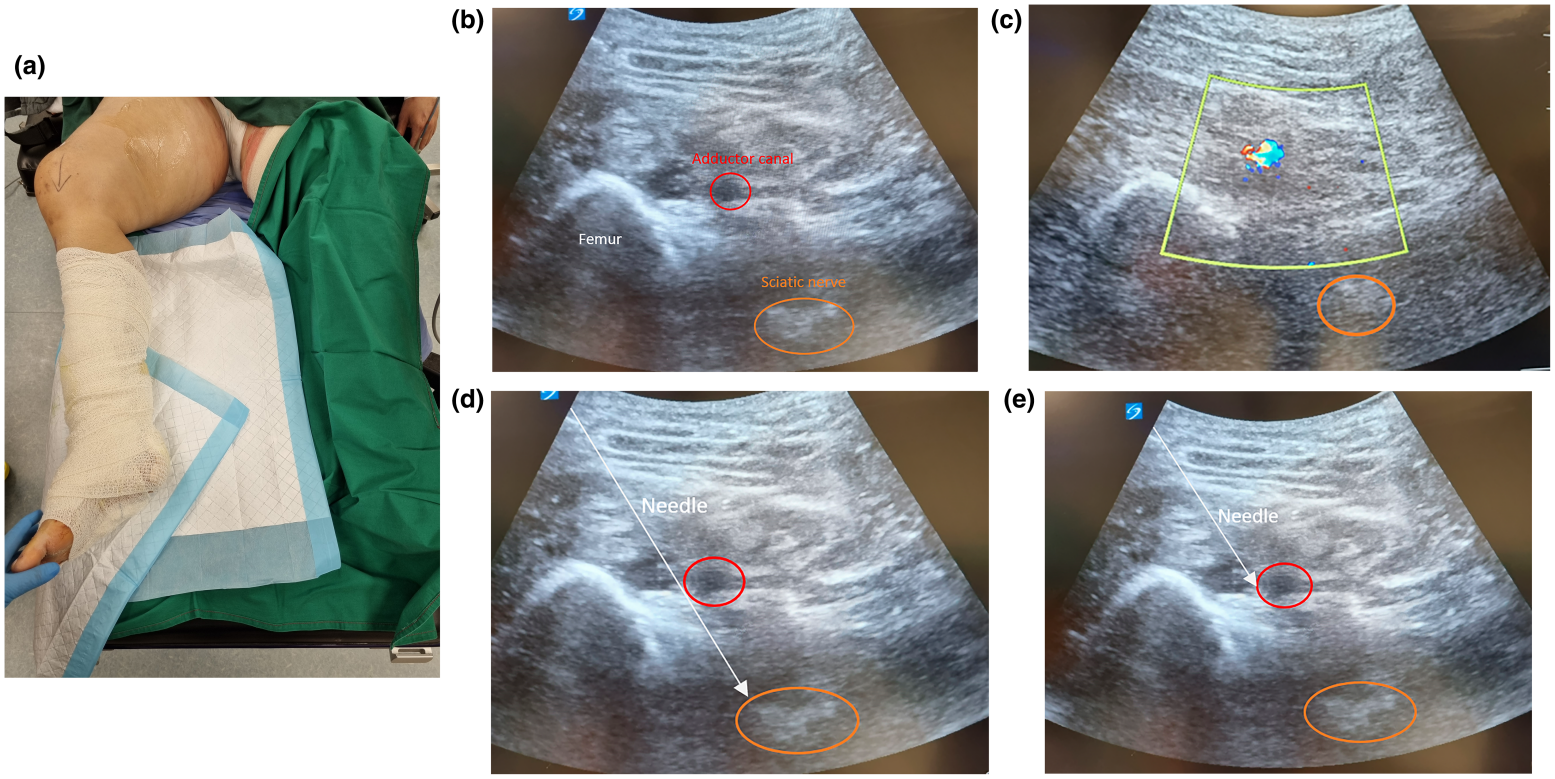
(a) Position of patient's right lower limb for sciatic and adductor canal block; (b) ultrasound imaging of both sciatic nerve and adductor canal; (c) colour Doppler of superficial femoral artery; (d) local anaesthetic deposited around the sciatic nerve; (e) local anaesthetic deposited in the adductor canal.
